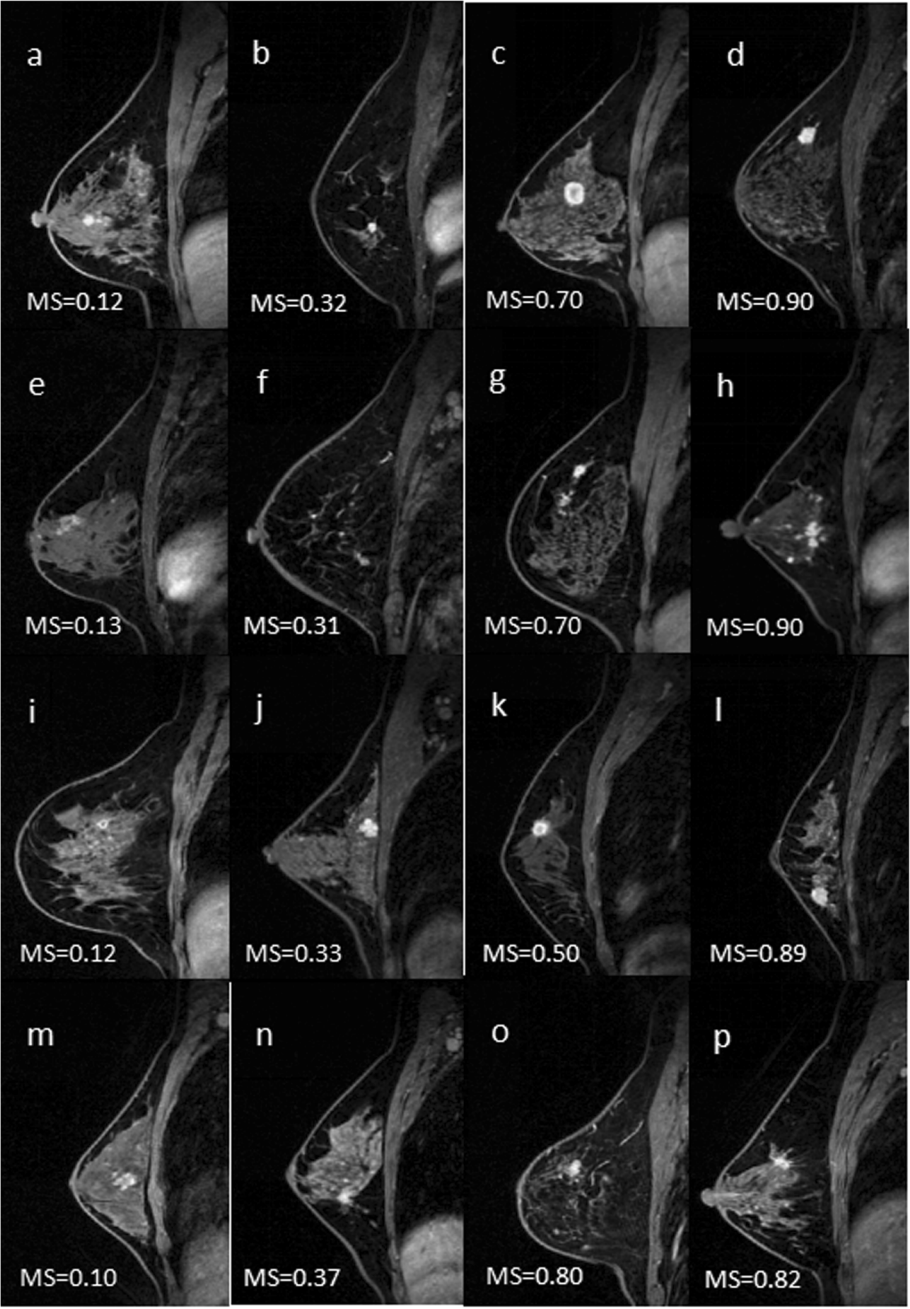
Some representative breast MRI studies from the independent consecutive test set as classified by the trained MRI radiomic signature. (a-d) Malignant mass examples; (e-h) Malignant non-mass examples; (i-l) Benign mass examples; (m-p) Benign non-mass examples
